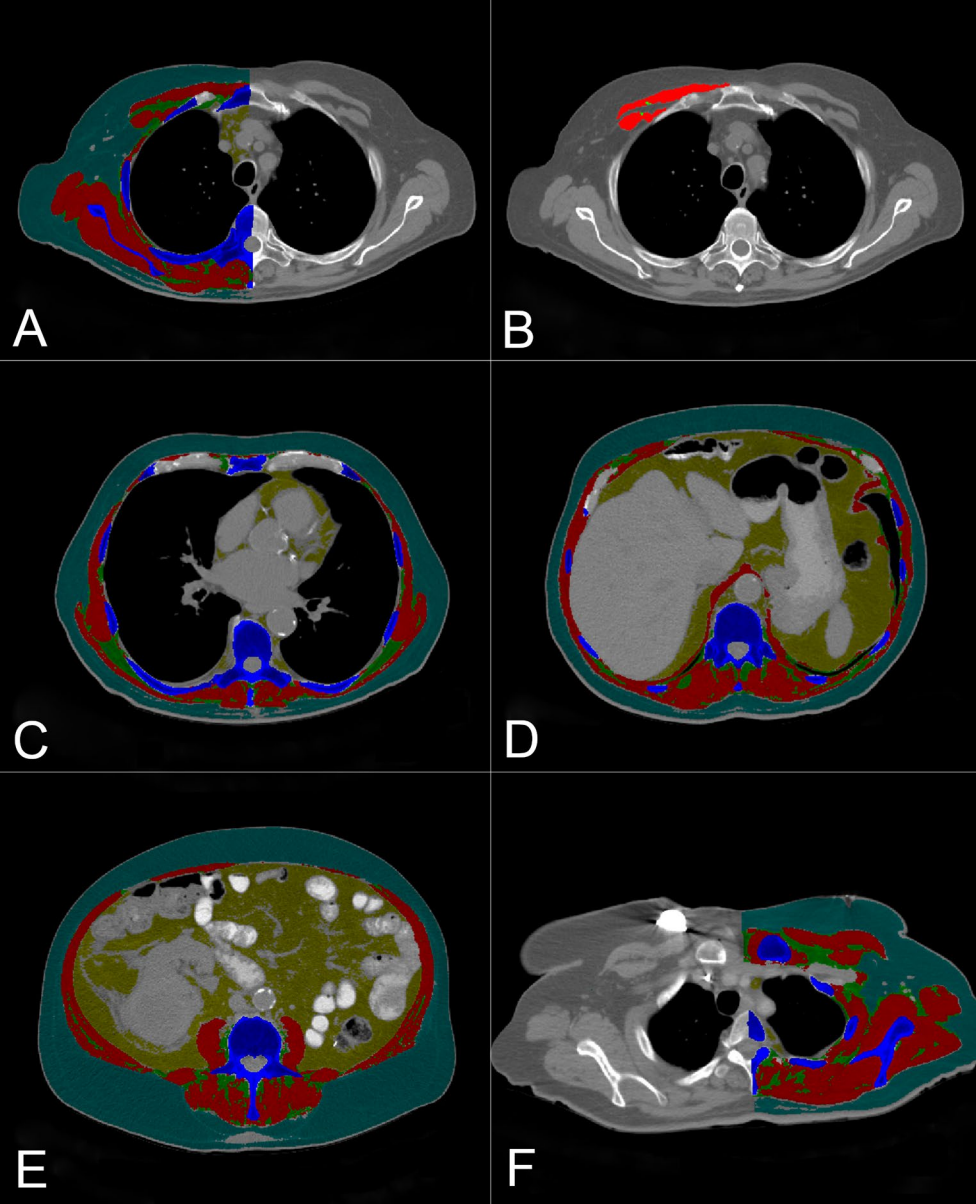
Tagged computed tomography axial images above the aortic arch (AbvAoAr; (A), unilateral total; (B), unilateral pectoralis) and at the eighth thoracic (T8; C), twelfth thoracic (T12; D), and third lumbar (L3; E) vertebrae. Measurements AbvAoAr were made unilaterally on the right unless a right-sided device was present (F). The tagged tissues were skeletal muscle (red), intermuscular adipose tissue (green), subcutaneous adipose tissue (teal), and visceral adipose tissue (yellow)
